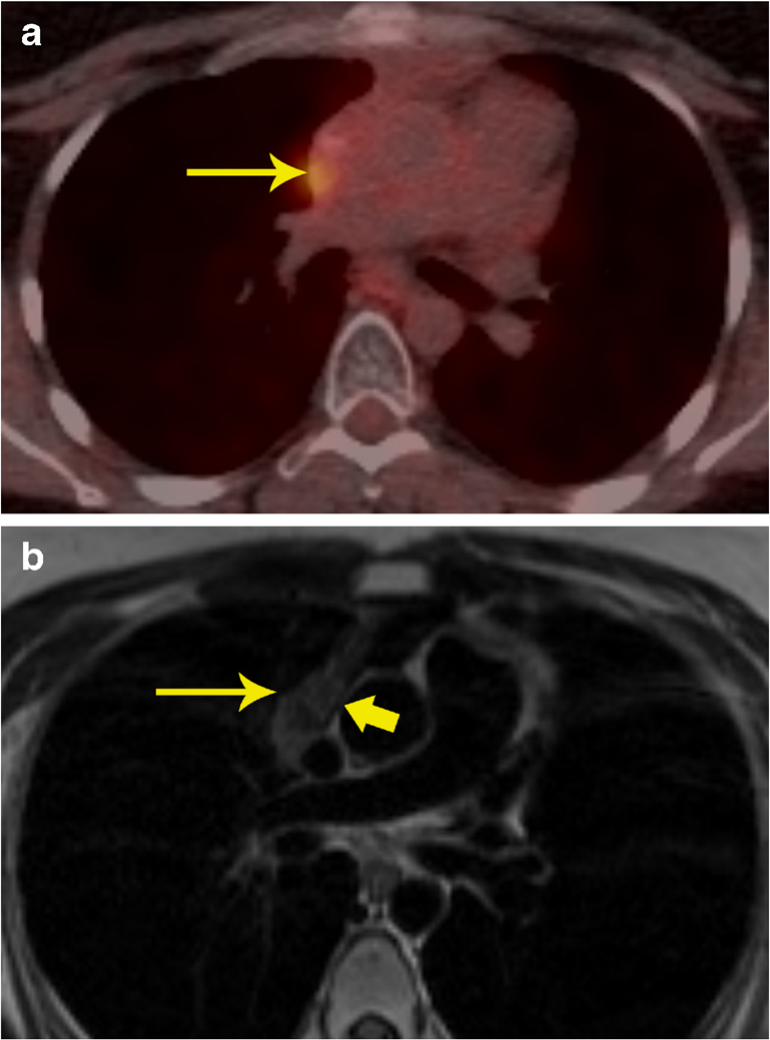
Evaluation of cardiac infiltration. a FDG PET/CT in a patient with lymphoma shows a focus of hypermetabolism adjacent to the SVC. The exact extent of this is not evident in the PET or the concomitant low-dose CT scan. b MRI was performed for evaluating cardiac extension. T1-weighted axial image shows that the mass (thin arrow) has a clear fat plane (thick arrow) with the SVC and cardiac structures, indicating that it is not infiltrating the cardiac structures
